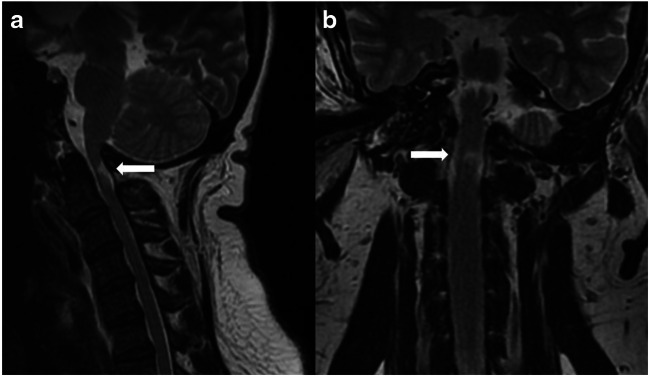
Sagittal (a) and coronal MRI (b) of the occipito-cervical region in a 28-year-old girl with achondroplasia. In spite of the mild stenosis of the foramen magnum on both antero-posterior and latero-lateral diameter, an atrophic damage of the upper cervical spinal cord can be appreciated (arrows)
